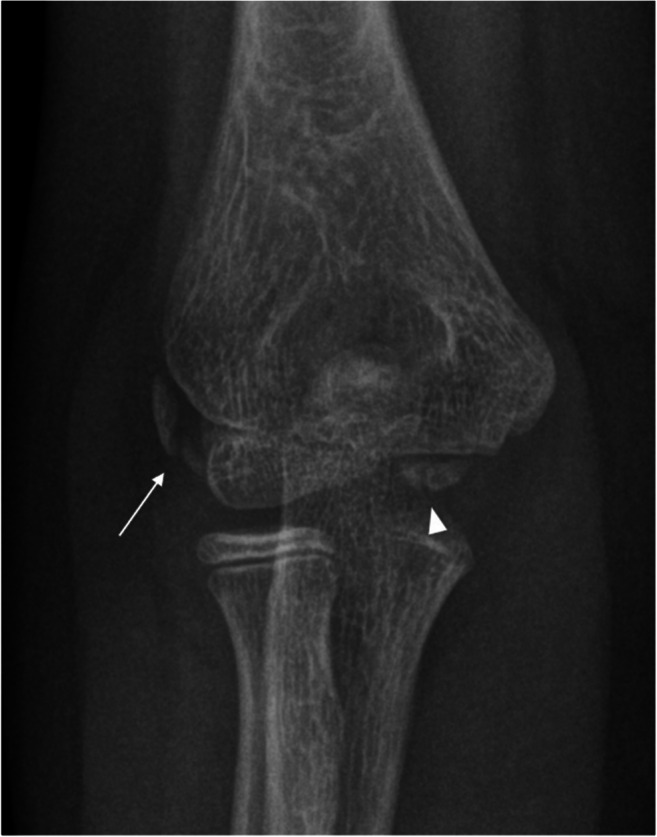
Radiograph of the right elbow of a 10-year-old boy in anteroposterior projection showing a multipartite lateral epicondyle consisting of two portions (arrow). The trochlear apophysis is typically multipartite and in this case consists of at least two parts (arrowhead)
